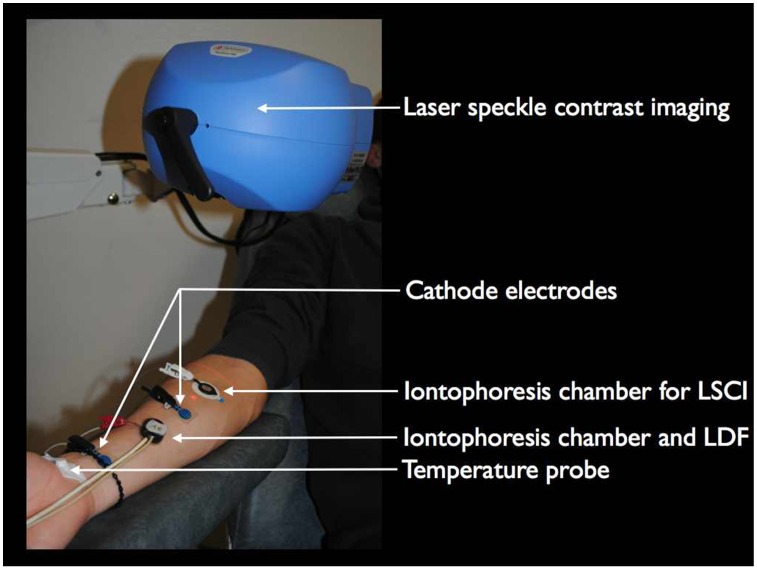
Typical recording using laser Doppler flowmetry (LDF) and laser speckle contrast imaging (LSCI) during protocol 1 and protocol 2.
